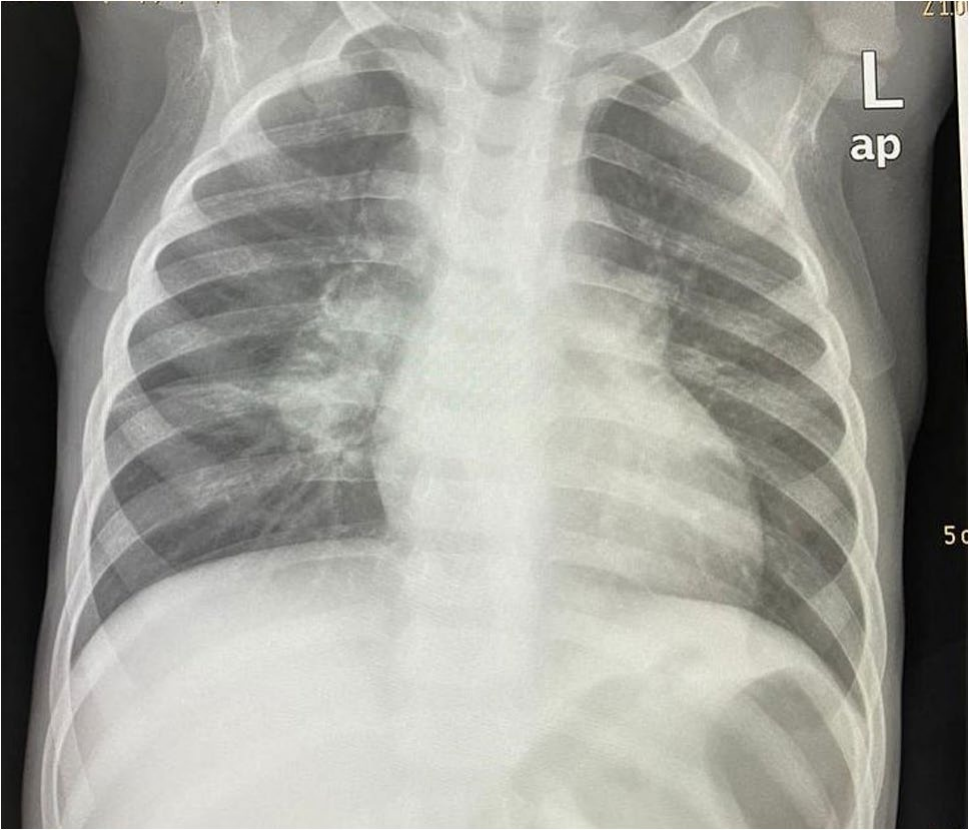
Anteroposterior chest radiograph in a 1-year-old boy with tuberculosis demonstrating bilateral hilar lymphadenopathy
